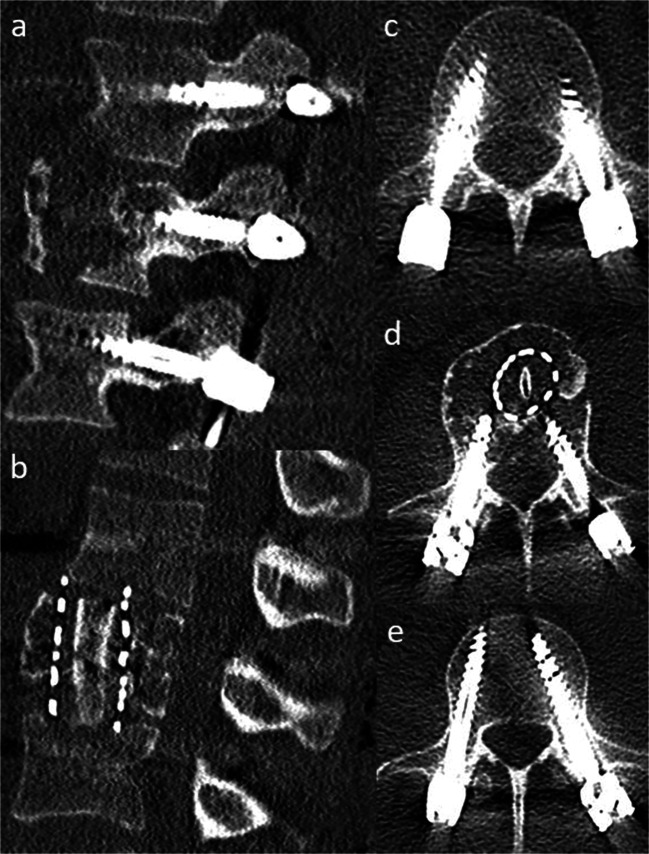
a, b Sagittal CT scans after a 360° approach with partial corpectomy, mesh cage, rib graft, and pedicle screw instrumentation. c–e Postoperative axial CT scans demonstrating pedicle screws in L1 (c), L2 (d), and L3 (e)
